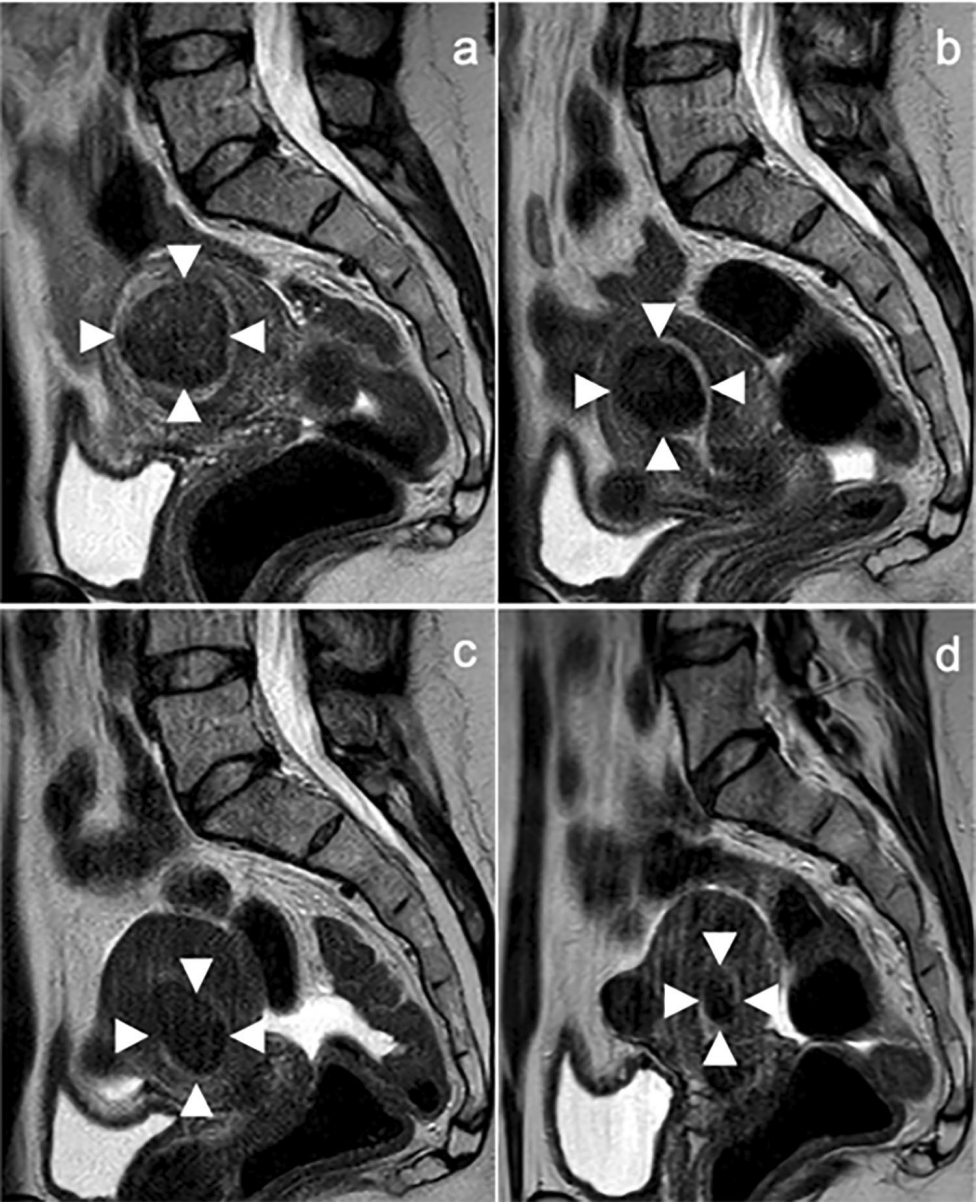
MRI-images (sagittal T2-weighted sequences) of a 41-yearS old premenopausal patient with multiple fibroids who presented with severe fibroid-associated symptoms (hypermenorrhea, dysmenorrhea, anaemia, pelvic pain and pressure). There was no fibroid-specific previous therapy. The largest fibroid was located in the fundus of the uterus and was the main target of ablation. In the 9-month follow-up a significant reduction of tumor volume was observed. (a) Large predominant target myoma in the uterine fundus before HIFU treatment. (b) At 3-month follow-up the volume reduction was about 53.7%. (c), (d) Fibroid volume reduction of about 87.8% after 6 months (c) and 92.7% after 9 months (d) post-HIFU.
